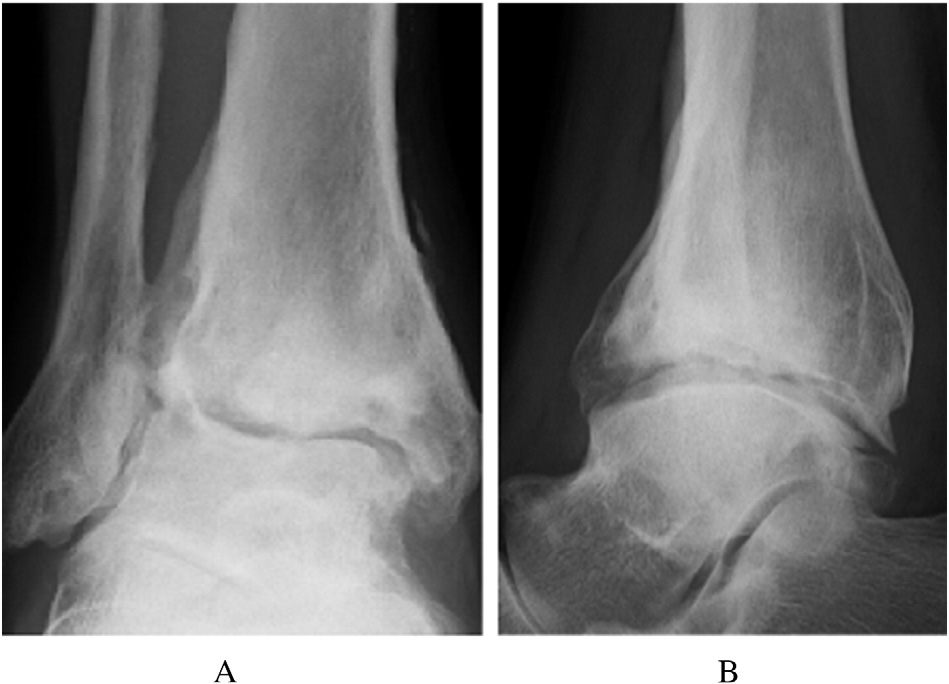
Radiographs obtained at 3 years after surgery showed an osteophyte in the anterolateral corner of the right plafond that was larger than that evident in the radiographs taken before OAT; forward displacement of the talus was not seen, and the joint space was preserved: (A) anteroposterior view, (B) lateral view.
